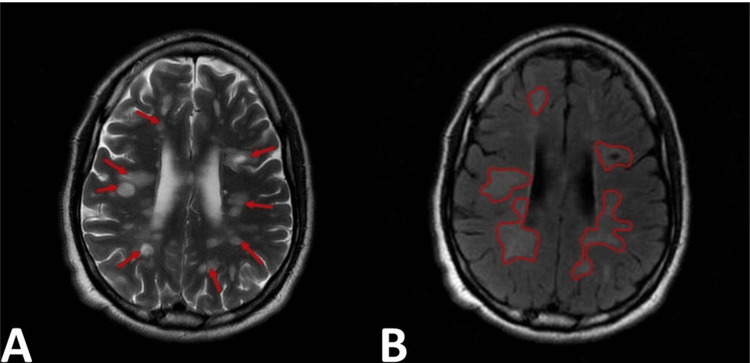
Axial T2 MRI of the brain at the level of the ventricles: (A) innumerable patchy foci of bright signal intensity throughout the white matter (red arrows); (B) patchy foci of edema associated with the lesions (outlined). MRI, magnetic resonance imaging
